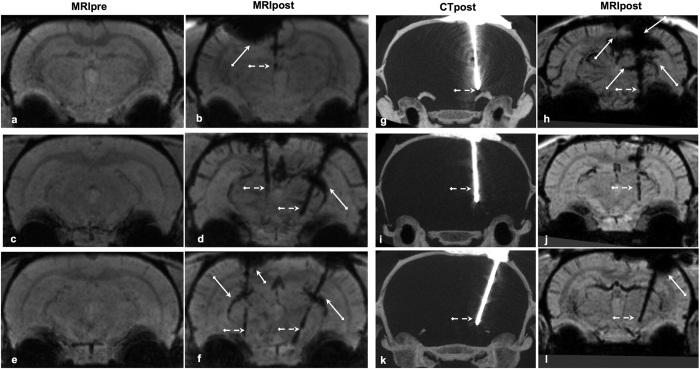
Surgery-related adverse effects visualized in post-operative MRI. Representative mIPs of pre-operative (column 1) MRI, mIPs of post-operative MRI (column 2, 4) and MIPs of post-operative CT (column 3 – maximum intensity projections) generated from coronal cross-sections covering the extent of the electrode trajectory. When compared to the pre-operative MR image (a,c,e), the post-operative images (b,d,f) show specific hypo-intense contrast along the trajectory (dotted white arrows), but often also a-specific hypo-intense clusters (indicated by continuous arrows) around the cortex (near electrode entry position) or superior colliculus vessel (radial to the trajectory), which appear to be likely indications of ICH or other deleterious effects of the surgery. As expected, post-operative CT in column 3 (g,i,k) does not show soft tissue contrast from the brain as well as any signs of deleterious effects as noted in the corresponding post-operative MRI (h,j,l).
